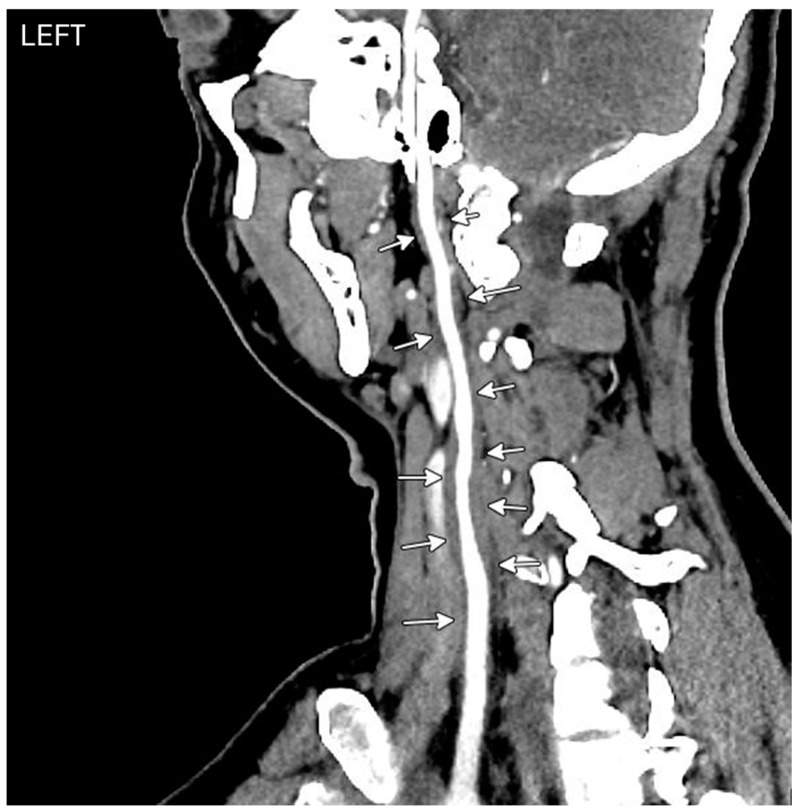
CT angiogram. Left carotid artery centerline reconstructions show diffuse arterial wall thickening (white arrows).
